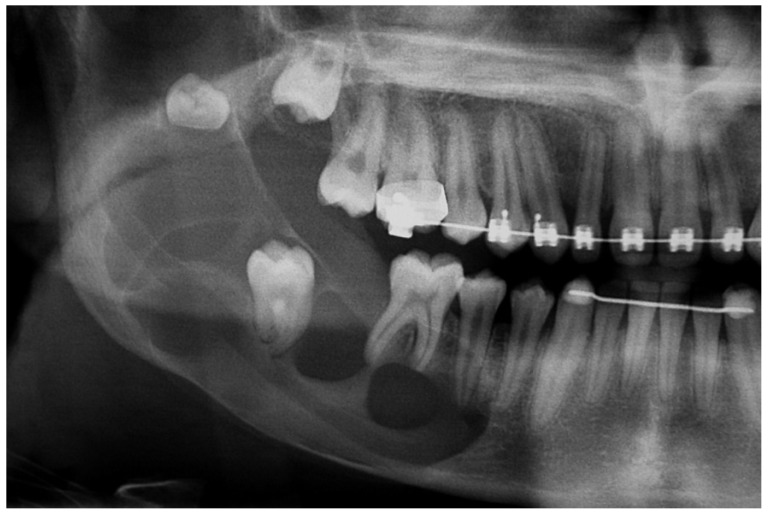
Panoramic radiography: A well-defined multiloculated radiolucent lesion involving impacted mandibular right second molar.
